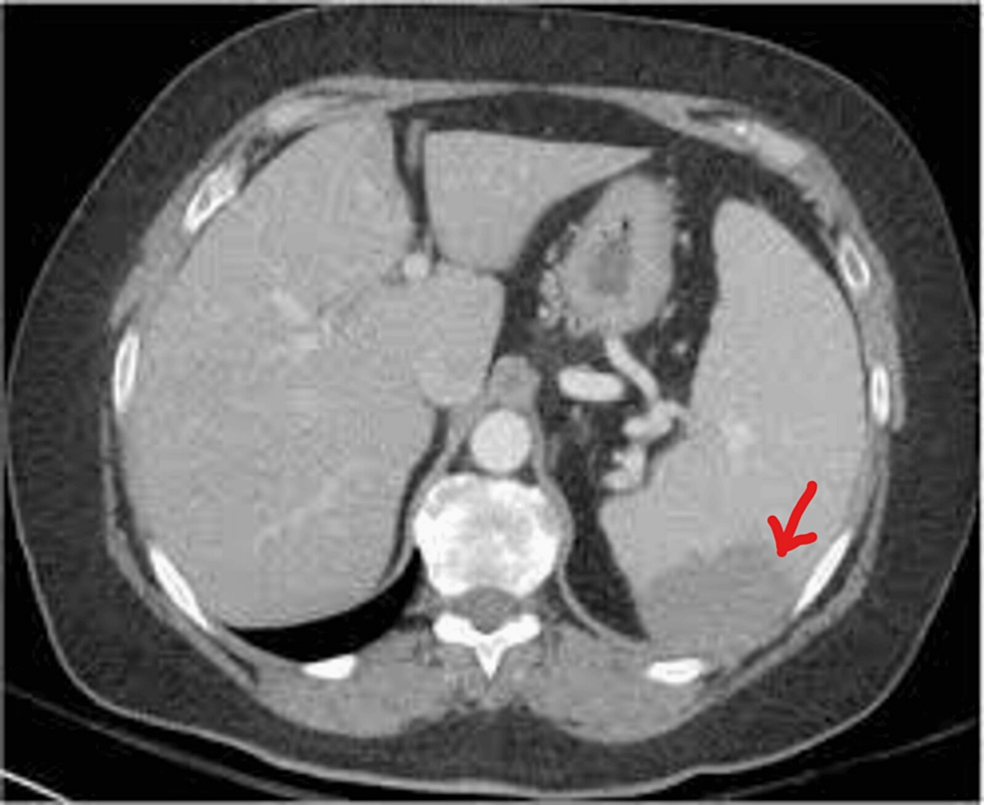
Abdominal CT showing an area of hypodense splenic infarct (as marked by red arrow)
